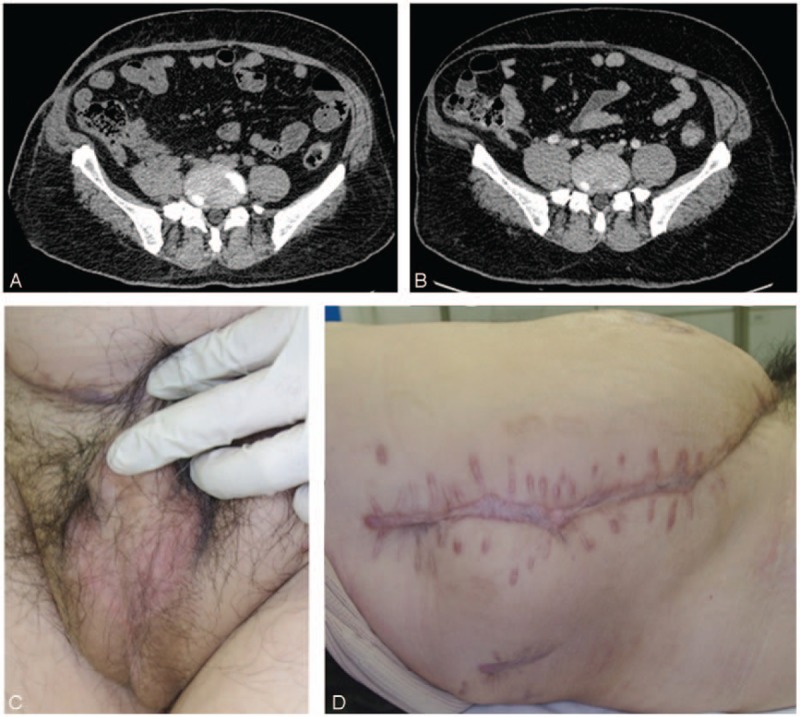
(A) Axial CT scan revealed that the abscess was partially absorbed on day 61, measuring 2.5 cm × 1.7 cm × 2.0 cm (vertical × anteroposterior × transverse). (B) Axial CT scan revealed that the abscess was completely absorbed 6 months later. (C) The appearance of the scrotum 6 months later. (D) The appearance of the flank 6 months later. CT = computed tomography.
